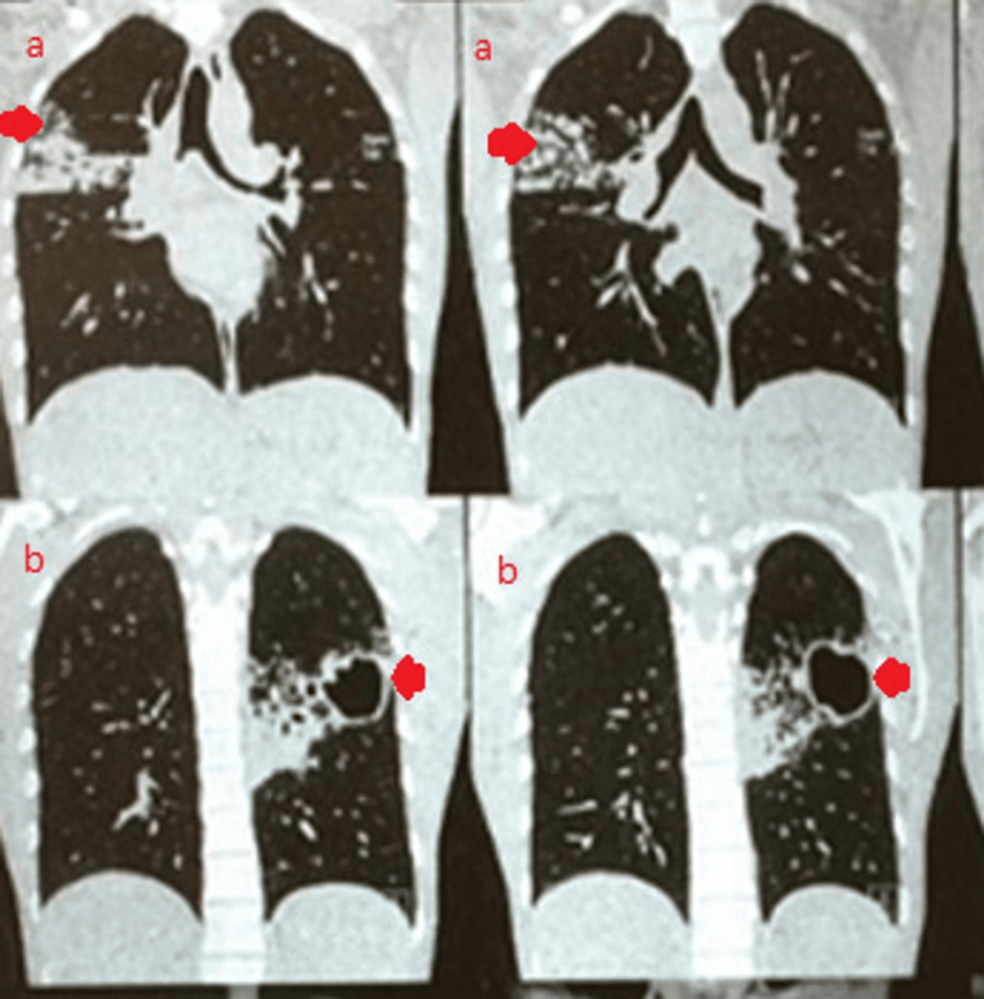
High-resolution computed tomography (HRCT) of the chest showing multiple coalescing cavitary lesions in the left upper lobe with peri-regional nodular and alveolar opacities and multiple coalescing nodular and alveolar opacities in the right upper lobe. (A) Multiple coalescing and alveolar opacities in the right upper lobe. (B) Multiple coalescing cavitary lesions in the left upper lobe with peri-regional and alveolar opacities.
